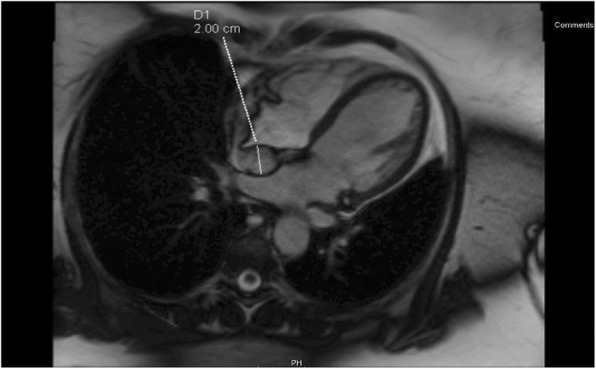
Transverse T2 weighted Cardiac MRI showing right atrial mass
